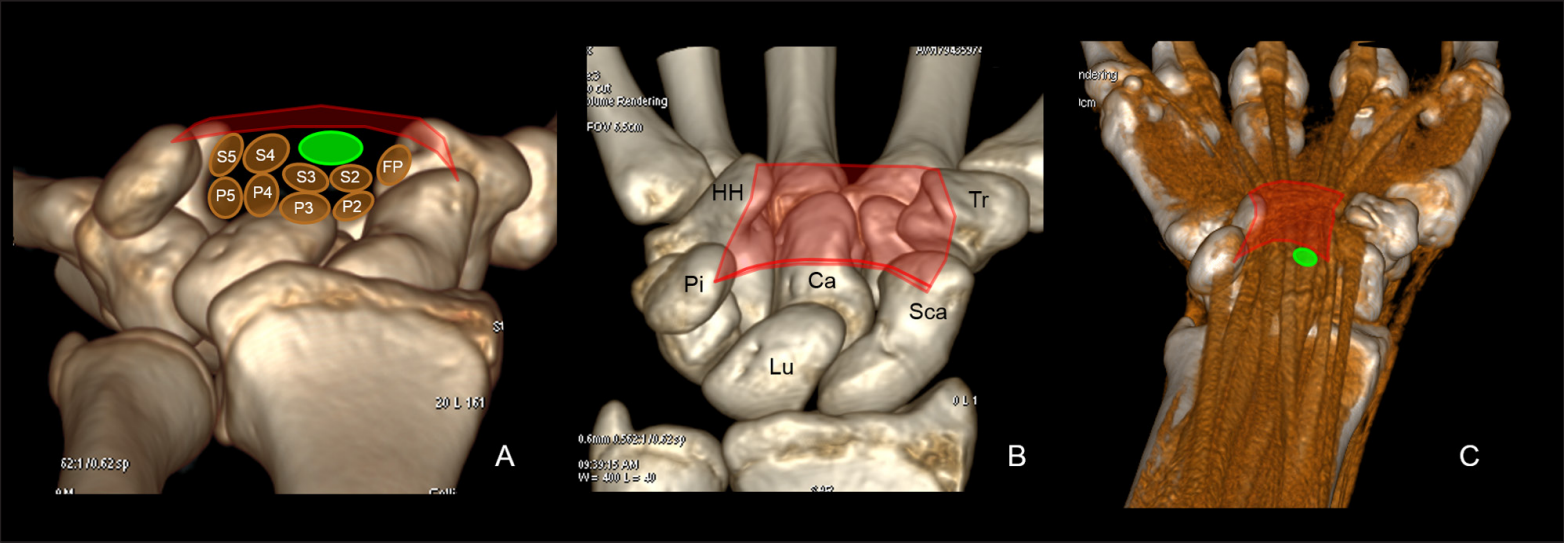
Normal anatomy of the carpal tunnel (A, B, C) Volume-rendered 3D computed tomography. Lu = Lunate, Ca = capitate, Sca = scaphoid, Pi = pisiform, Tr = Trapezium, HH = Hamate Hook, FP = flexor pollicis longus muscle, P = flexor digitorum profundus, F = flexor digitorum superficialis (1, 2, 3, 4) muscle. The osteofibrous canal is situated in the volar wrist and is closed anteriorly by the flexor retinaculum (red) extending from the scaphoid (Sca) and pisiform (Pi) to the trapezium (Tr) and the hook of the hamate (HH). The carpal tunnel contains nine tendons: the flexor pollicis longus (FP), the four flexor digitorum superficialis (S) and the four flexor digitorum profundus tendons (P). The median nerve (green) reaches the carpal tunnel and travels through it in a superficial position just below the flexor retinaculum and above the flexor tendons of the index and middle fingers.
